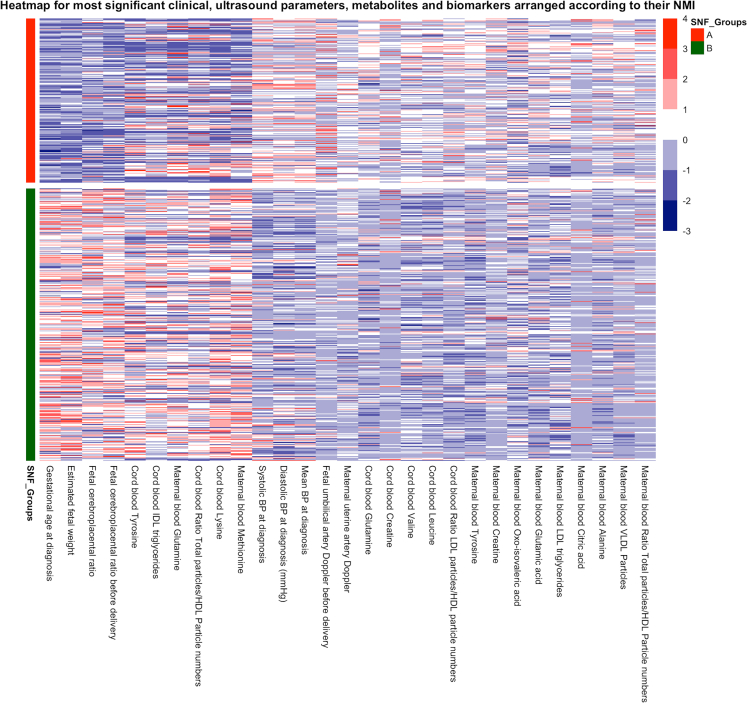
Heatmap including principal clinical, ultrasonographic, and metabolomic signatures in each phenotype The heatmap shows the 54 most variable features among the two SNF clusters (same color as Figure 3), with each column representing a feature. As shown on the scale, dark blue indicates a high concentration of a particular feature in a specific group compared to other groups.
